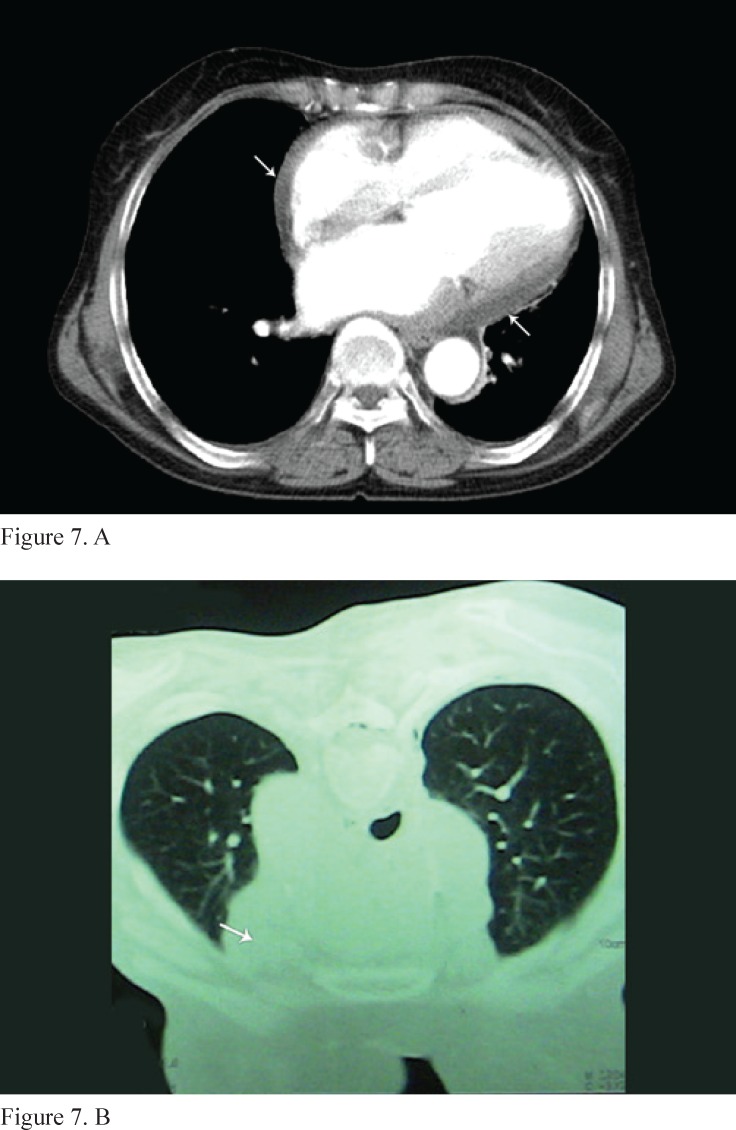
Chest computed tomography scan, without (A) and with (B) contrast, shows a severely thickened pericardium (arrow).
